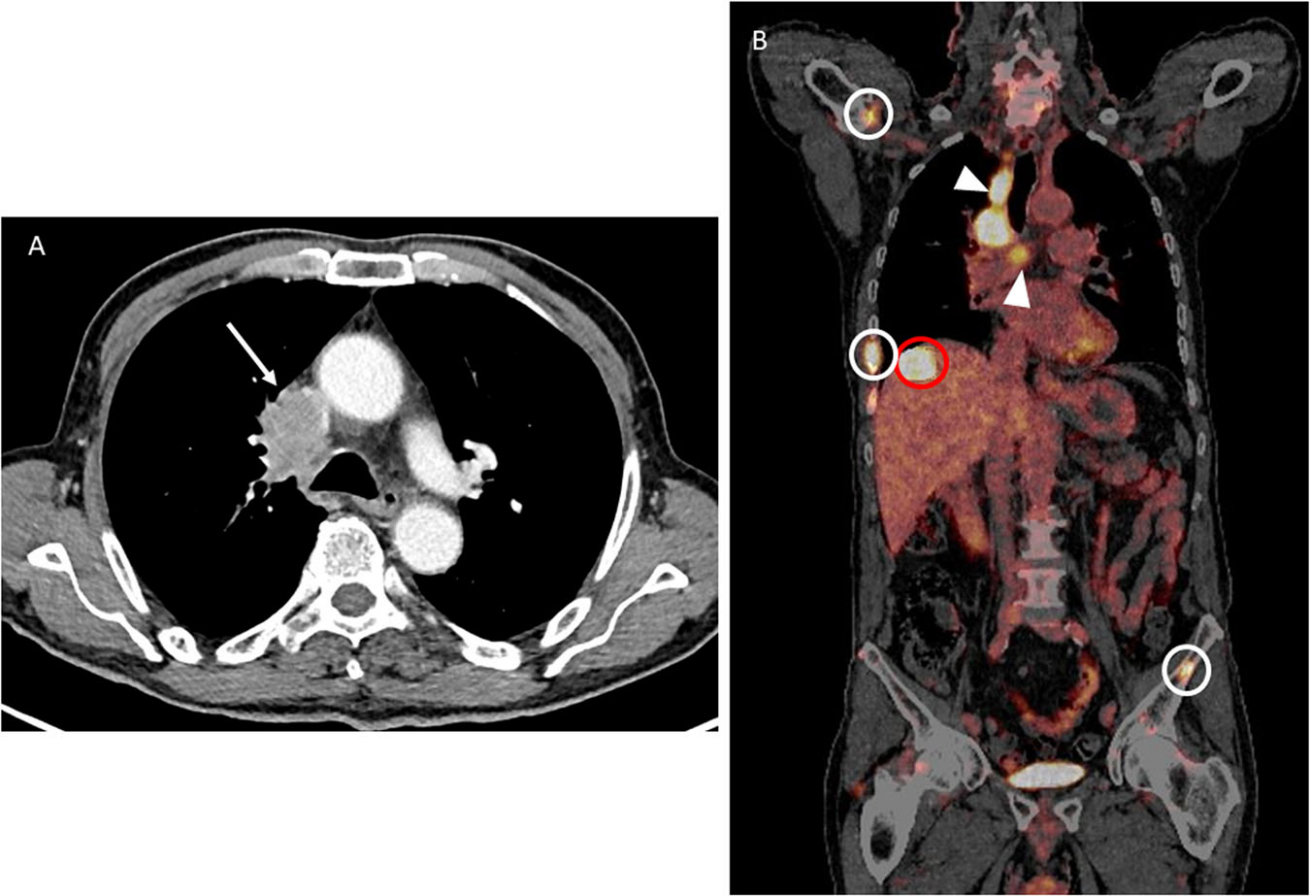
A 58-year-old male with a lung adenocarcinoma infiltrating the superior vena cava (T4) (white arrow in A). PET-CT (B) shows ipsilateral lymphadenopathies (white arrowheads) in superior paratracheal and subcarinal stations (N2b), as well as metastases in the liver (red circle) and bones (white circle) (M1c2), with a subsequent stage IVB (no stage changes according to the 9th TNM)
